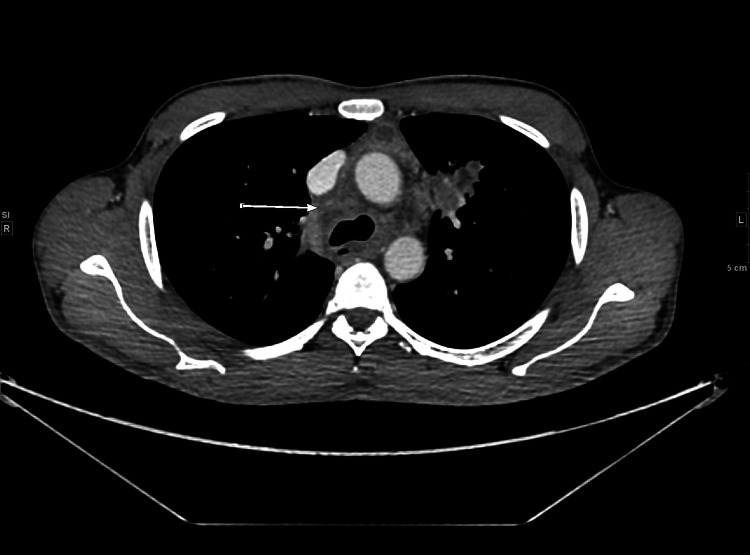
CT scan chest (axial view) showing interval increase in right paratracheal lymph node size This CT scan image shows that the paratracheal lymph node has increased to 18 mm in size from 9 mm in previous images when the patient was first treated.
